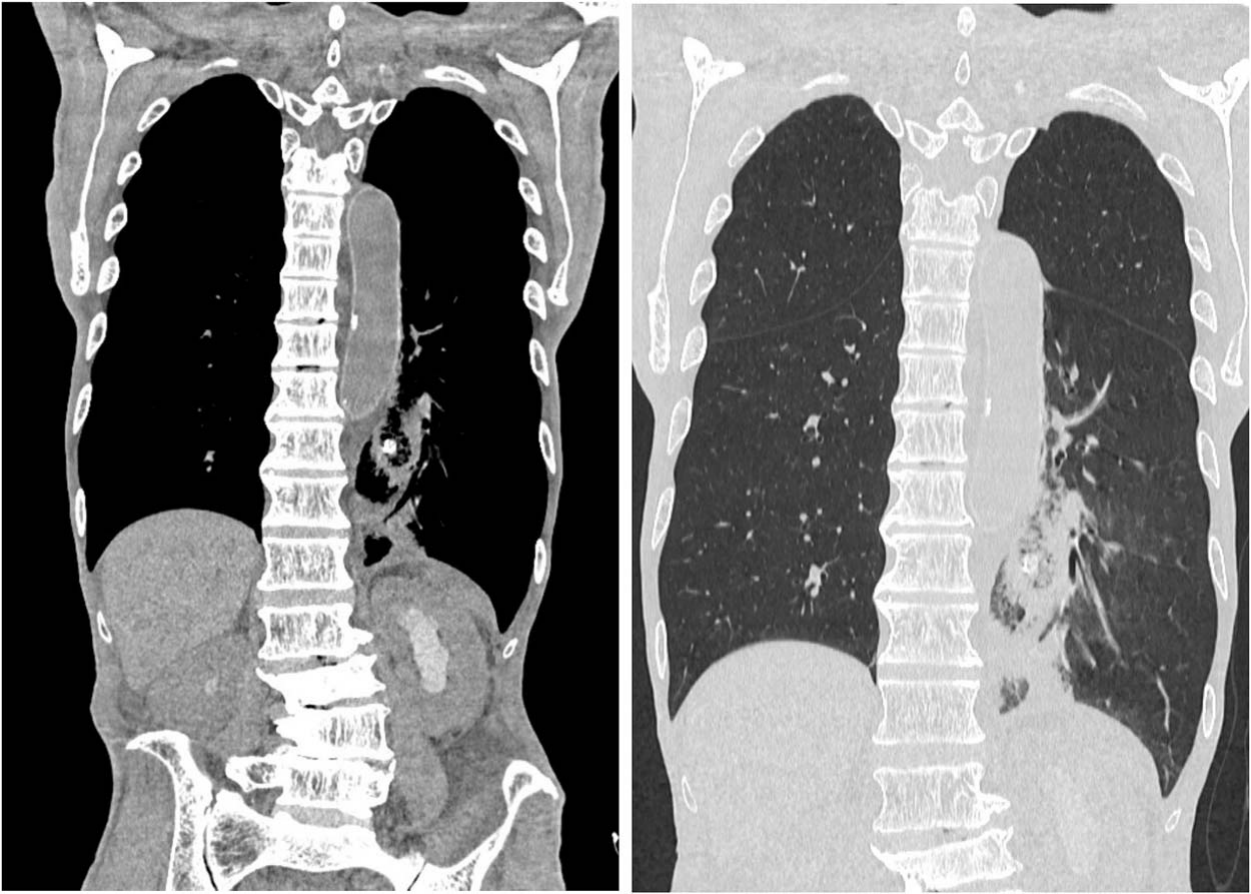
Postoperative drain migration. Chest and abdominal CT scan showing drain tip migration into the thoracic cavity.
